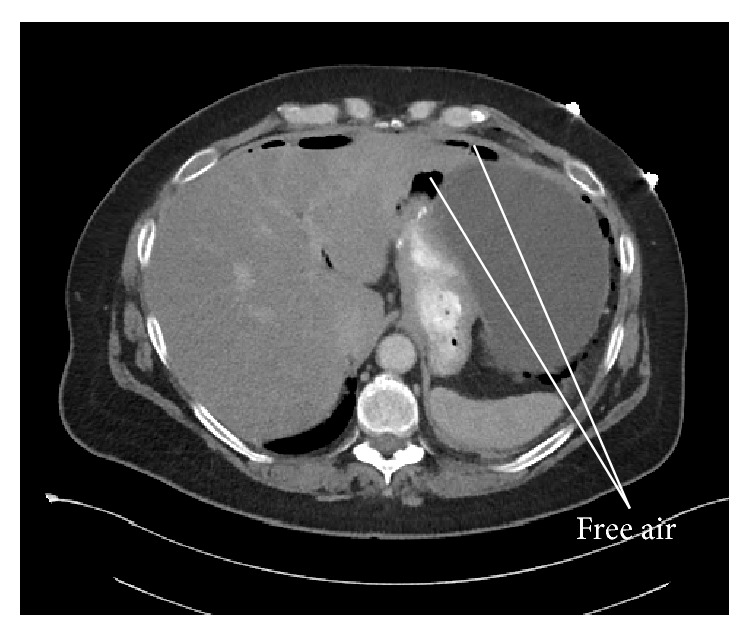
CT scan of abdomen showing moderate to large amount of intraperitoneal free air, likely representing bowel perforation.
